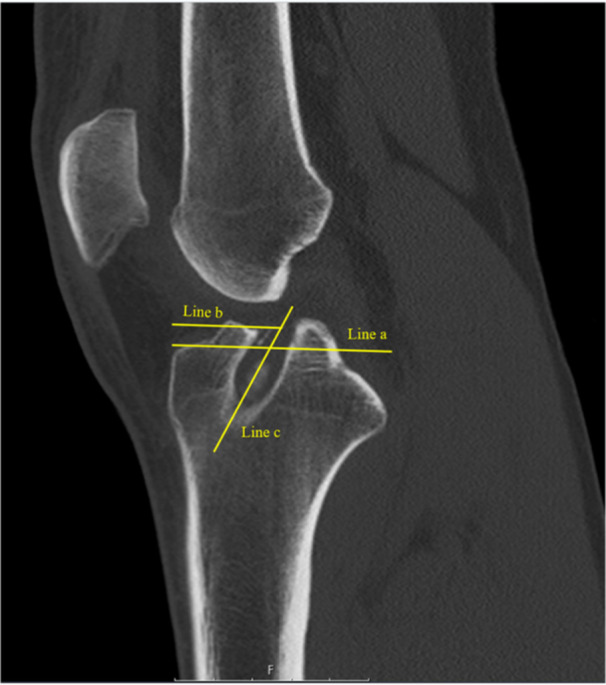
Determination of the location of the tibial tunnel on sagittal computed tomography [23]. (Line a) maximum anterior‐posterior diameter of the tibia (= 100%). (Line b) distance to the center of the tibial tunnel. (Line c) center of the tibial tunnel.
